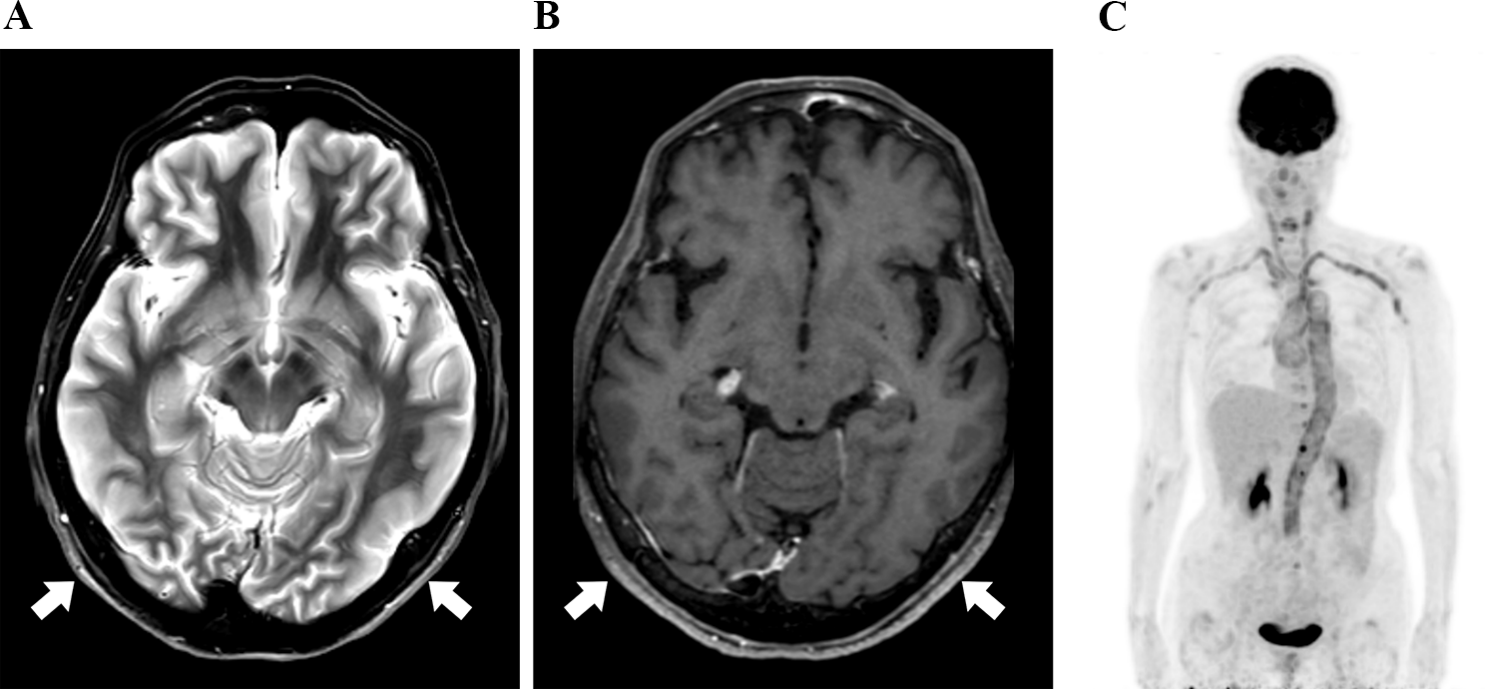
Case 14 (giant cell arteritis). (a), STIR image shows high signal intensity areas of posterior extracranial soft tissues. (b), Contrast-enhanced T1-weighted (CE-T1W) image shows weak enhancement areas of posterior extracranial soft tissues. (c), FDG-PET shows the FDG uptake of aortic wall and subclavian and common carotid arterial wall.
